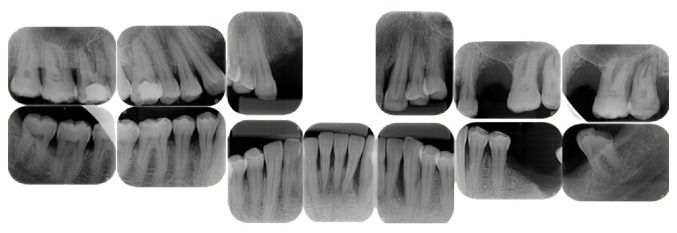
Radiographic features of severe periodontal disease. Generalized vertical and horizontal bone loss.
